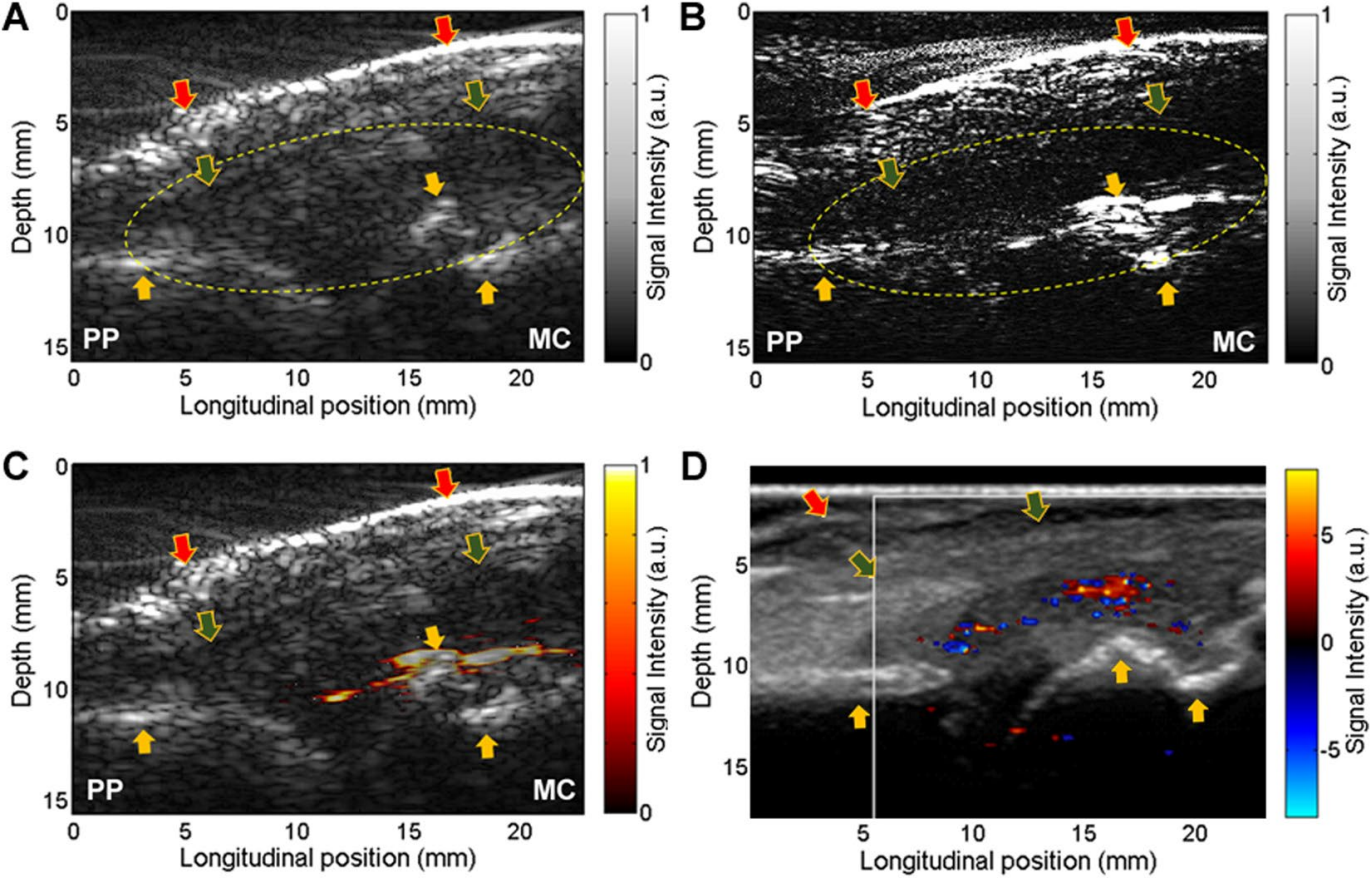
PA and US dual imaging of a right hand 2^nd^ MCP joint affected by inflammatory arthritis. PP: proximal phalanges. MC: metacarpals. (A) Gray scale B-mode US image. (B) Gray scale PA image of the same imaging plane. (C) Pseudo-color PA image superimposed on the gray scale US image, demonstrating the active vascularity in the joint. (D) US Doppler image acquired by a commercial US unit confirming the active synovitis in the studied joint. Yellow arrow: bone; Green arrow: tendon; Red arrow: skin.
